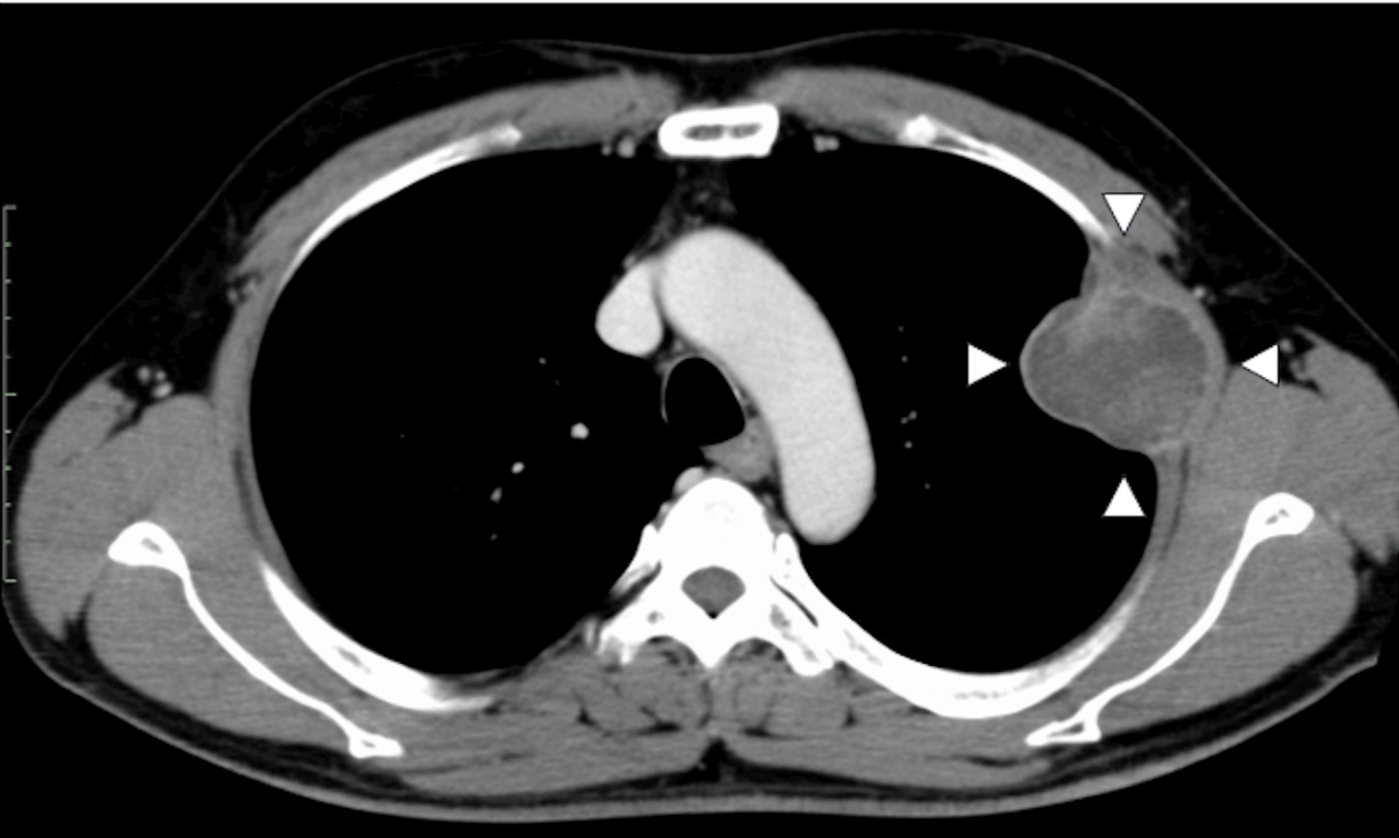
Preoperative CT scan Arrowheads indicate the tumor located inside and outside the thoracic cavity. CT: computed tomography
